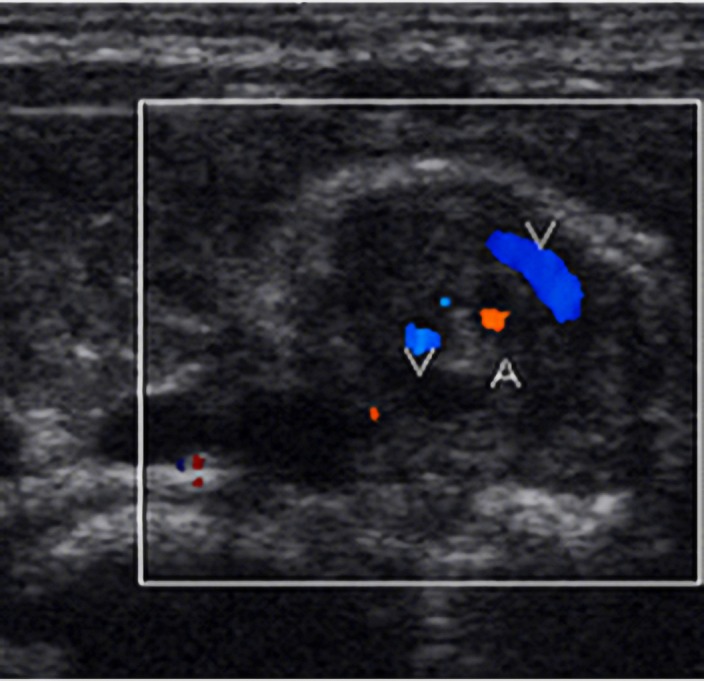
A 5-day male neonate with bilious vomiting Transverse color Doppler sonogram of the upper abdomen shows a whirlpool sign with a clockwise direction. Capital letter A indicates SMA and V indicate SMV.
